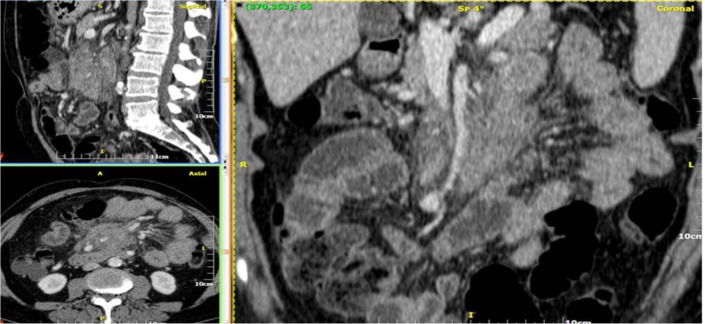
CT scan of the abdomen: paraaortic and mesenteric lymphadenopathy, hyperaemia/congestion of the mesenteric vessels, turbidity of the mesenteric fat, and enteritis.
